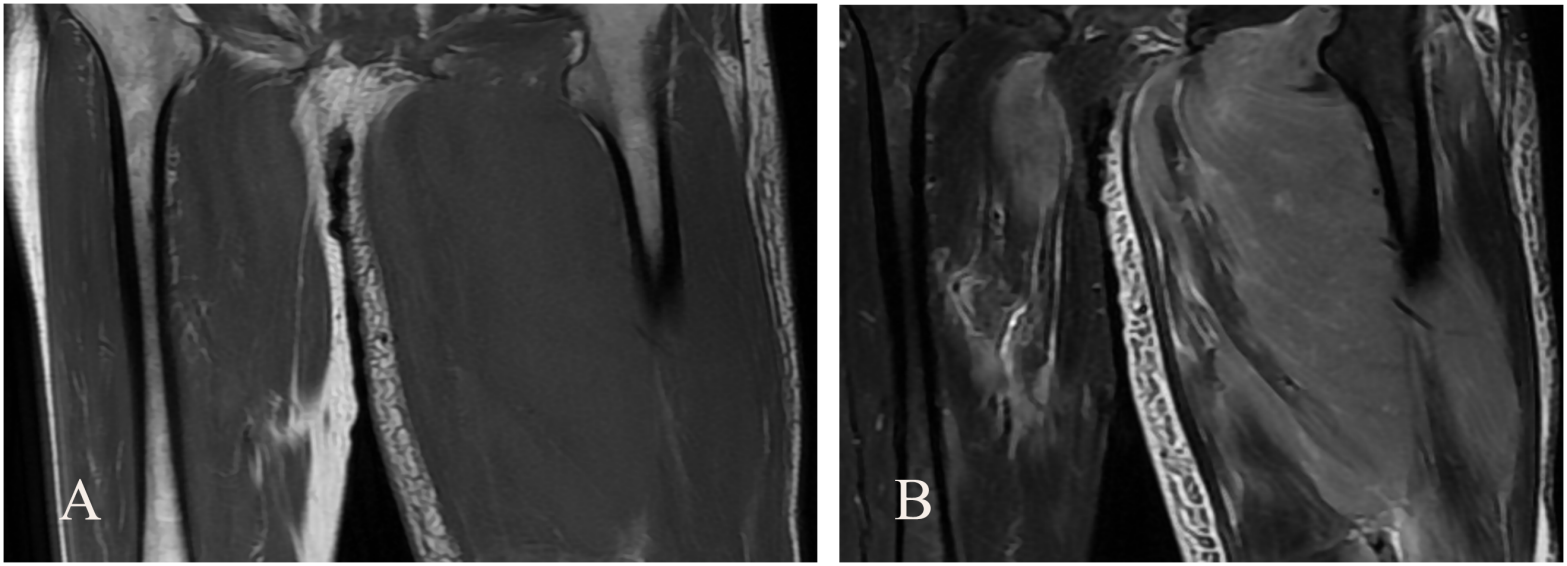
Coronal MRI images of lower extremities. (A) T1-weighted imaging (T1WI) sequence shows patchy long T1 abnormal low signal intensity in the middle and upper muscular layer of left thigh. (B) T2-weighted imaging (T2WI) sequence showed patchy long T2 abnormal high signal intensity in the middle and upper muscular layer of left thigh.
